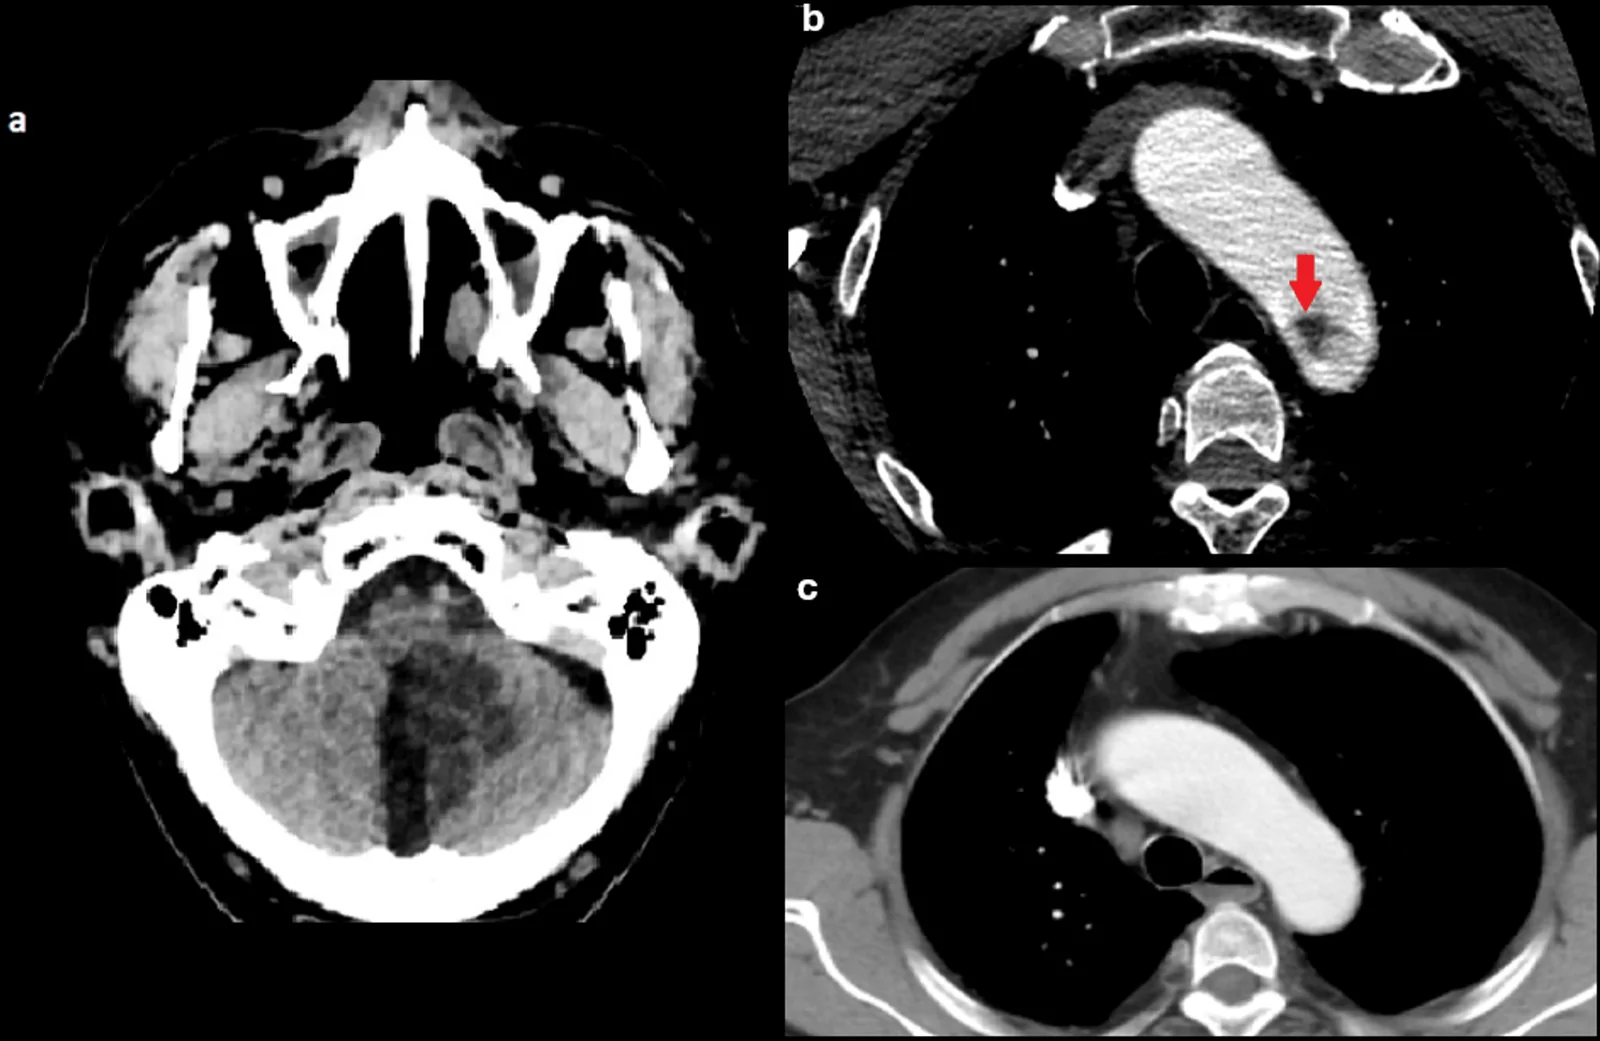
(a) CT scan image of a 63-year-old patient with an ischemic stroke in the left PICA territory, (b) CT scan image showing a floating thrombus of the aortic arch in the same patient, (c) follow-up CT scan image demonstrating complete thrombus resolution after 9 days of therapeutic anticoagulation with low molecular weight heparin (Case 6)
